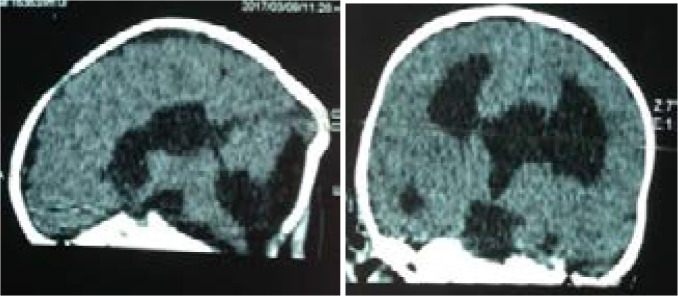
Brain CT without injection in sagittal and axial parenchymal window: left cortical and subcortical parafalcoreal hypodensity, associated with colpocephaly, complete agenesis of the corpus callosum and agenesis of vermis
